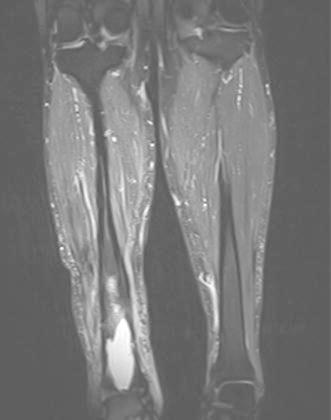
MRI appearances of chronic osteomyelitis of the right distal tibia
